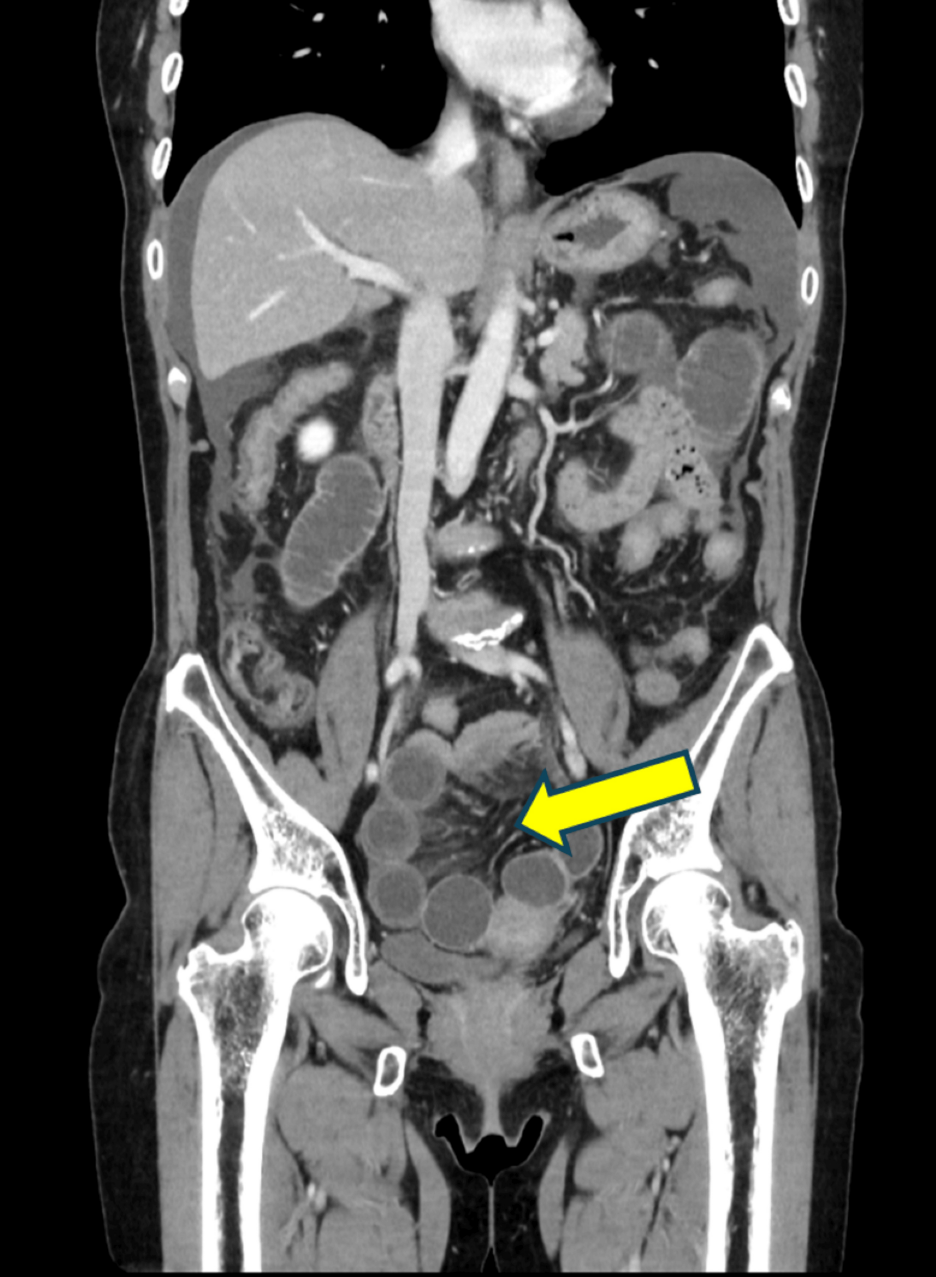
Contrast-enhanced abdominal CT revealed convergence of the mesentery near the uterus.
